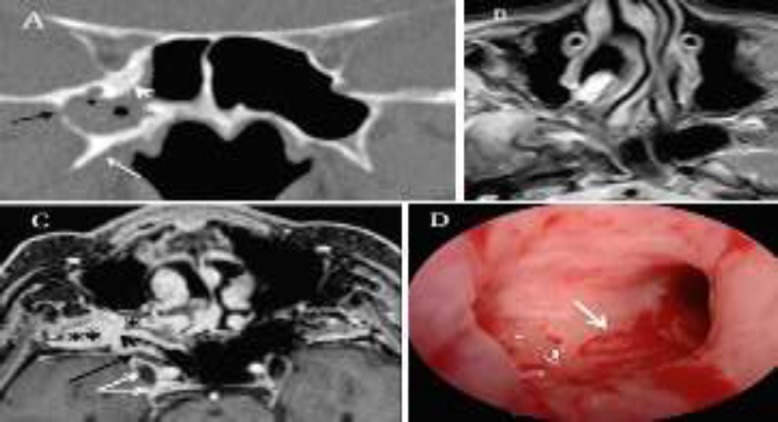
(A) Coronal CT scan shows an opacity of the lateral recess of the right sphenoid sinus (SS) with thickening and sclerosis of bone surrounding the pterygoid process (white arrow) and the orbital apex (white arrowhead). Focal interruption of the foramen rotundum (small star) and lateral recess of the sphenoid sinus is also present.(B) Axial T2-weighted MRI demonstrates a marked hypointense signal (small star) in the lateral recess of the SS with inflamed mucosa at the periphery. (C) Axial gadolinium enhanced T1-weighted MRI shows soft tissue protruding through the breach in the lateral recess wall of the SS (black arrowhead), invading the right internal pterygoid muscles, the masticatory fossa (double small star), and the pterygopalatine fossa (single small star) and infiltrating CN V2 (black arrow) and CN V3 (white arrow) trigeminal branches. Endocranially, the tissue was extended into the cavernous sinus surrounding the Gasser ganglion (double angled arrow). (D) Intraoperative view during endoscopic sinus surgery indicates the erosion of the lateral recess wall of the right SS (white arrow)
